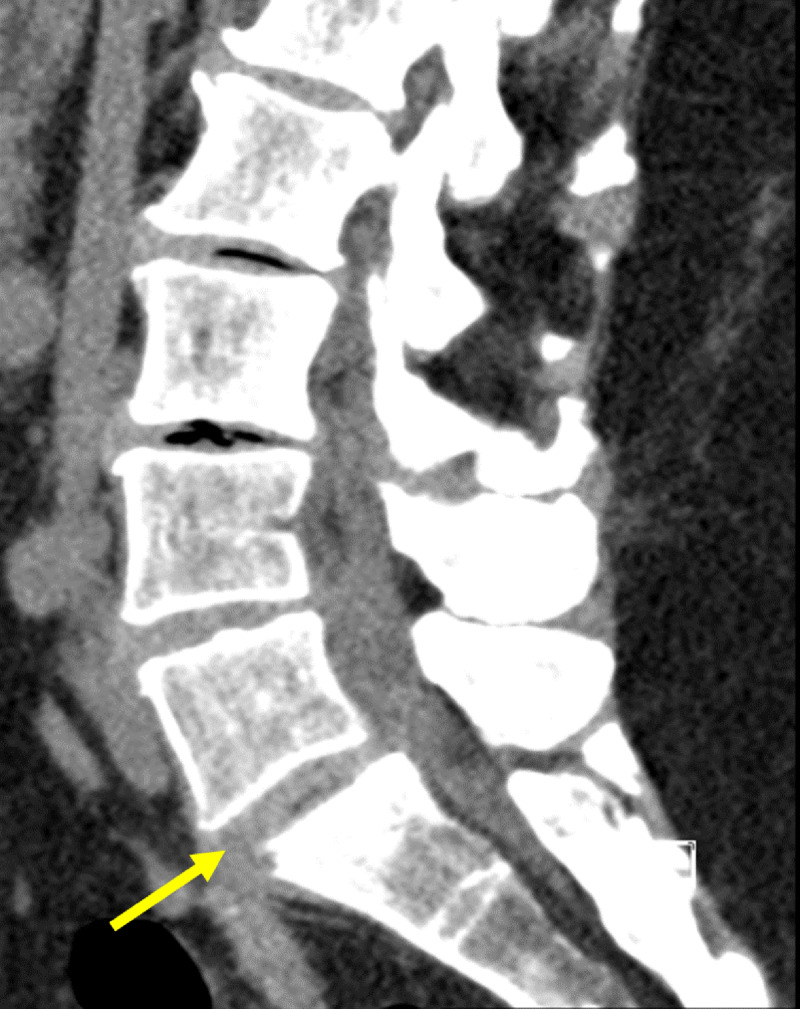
Sagittal non-contrast CT of lumbar spine: Initial CT-scan showing collection from L5-S1 disk with fistulation downwards, an anterolisthesis of L5-S1 with inter-apophysary posterior arthritis and a compression of the right root of S1 by the intervertebral disk.
